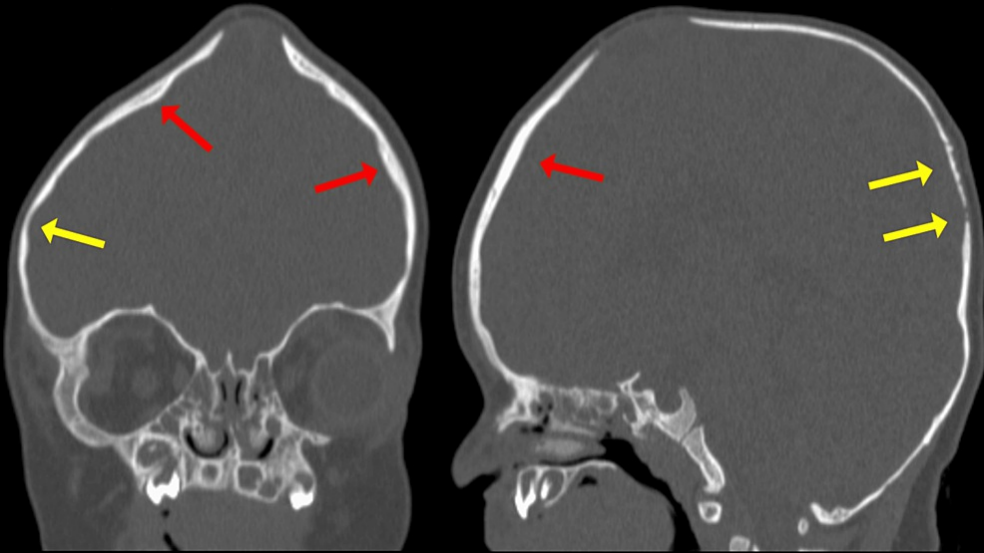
The head computed tomography (CT) scan revealed regions where skull bones varied in thickness (thick – red arrow, thin – yellow arrow).
